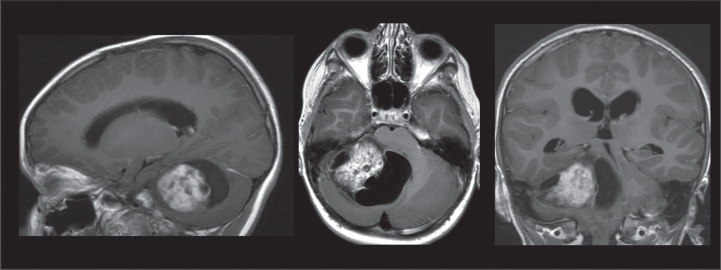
MRI findings of a tumor in the right cerebellar hemisphere. T1 weighted sequences in a sagittal, axial, and frontal view.
